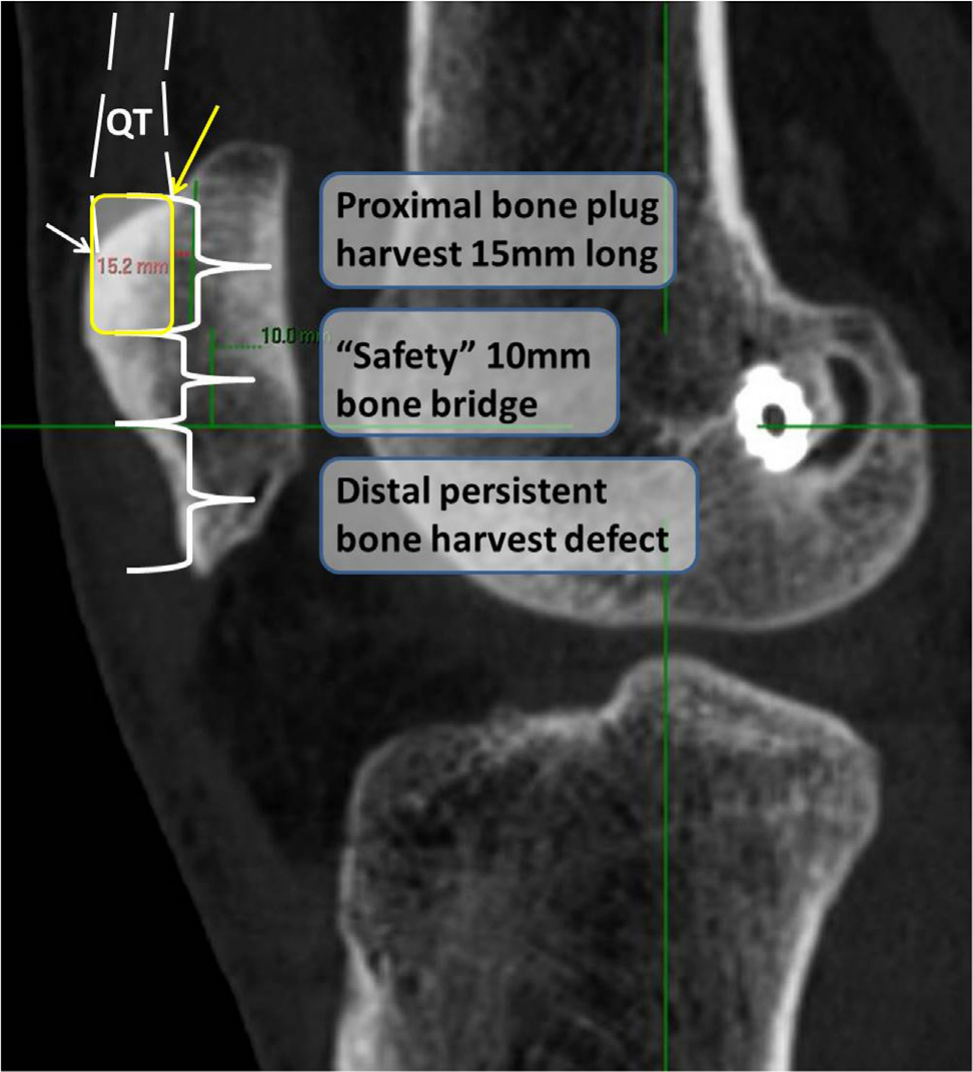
Two-dimensional sagittal computed tomography cut of a left knee through the center of the distal bone defect. Preoperative key measurements are performed for harvesting of a minimum 15-mm-long proximal patellar bone plug and ensuring an 8- to 10-mm “safety” bone bridge is remaining between the distal and proximal bone plug harvest sites. For this measurement, it is crucial to identify the deep insertion of the quadriceps tendon (yellow arrow) as the most proximal bony edge, which allows 5 mm or more of additional bone plug length compared to the superficial patella insertion of the quadriceps tendon (white arrow) and assists in avoiding cutting too distally when preparing the proximal patella bone plug.
